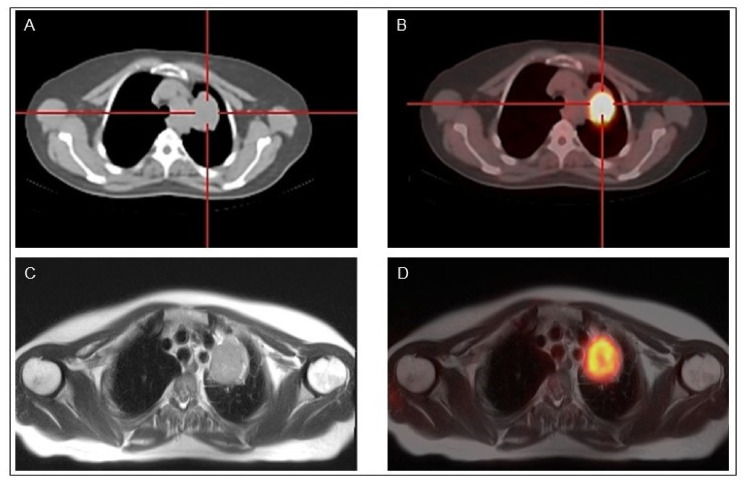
Classical papillary thyroid cancer in a 45-year-old man^18^F-FDG PET-CT (A,B) and ^18^F-FDG PET-MR (C,D) show a mediastinal lymph node with high ^18^F-FDG uptake.
